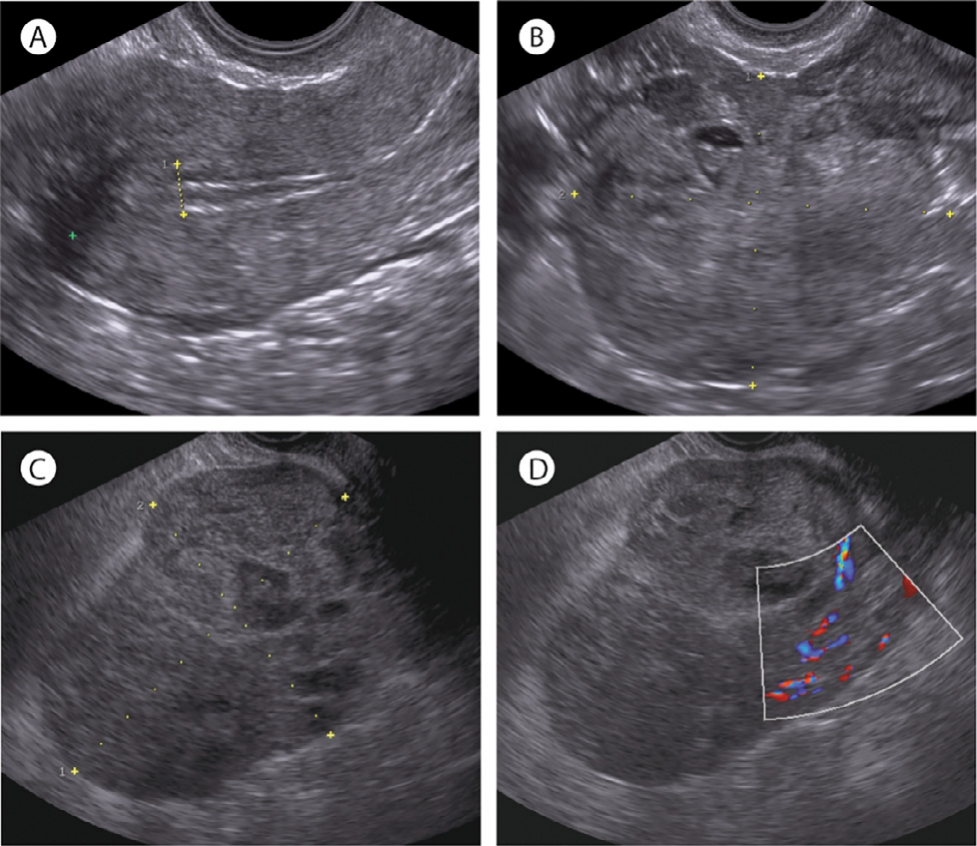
Images made during transvaginal ultrasonography at first presentation (A-B), showing a normal uterus without intracavitary abnormalities (A), and a right adnexal mass of 8×7cm (B). Images made during transvaginal ultrasonography at presentation at the gynecologic emergency unit (C-D), showing the enlarged right adnexal mass of 12×9cm with both solid and cystic components (C), and flow on color Doppler imaging (D). Yellow dotted lines indicate measurements.
